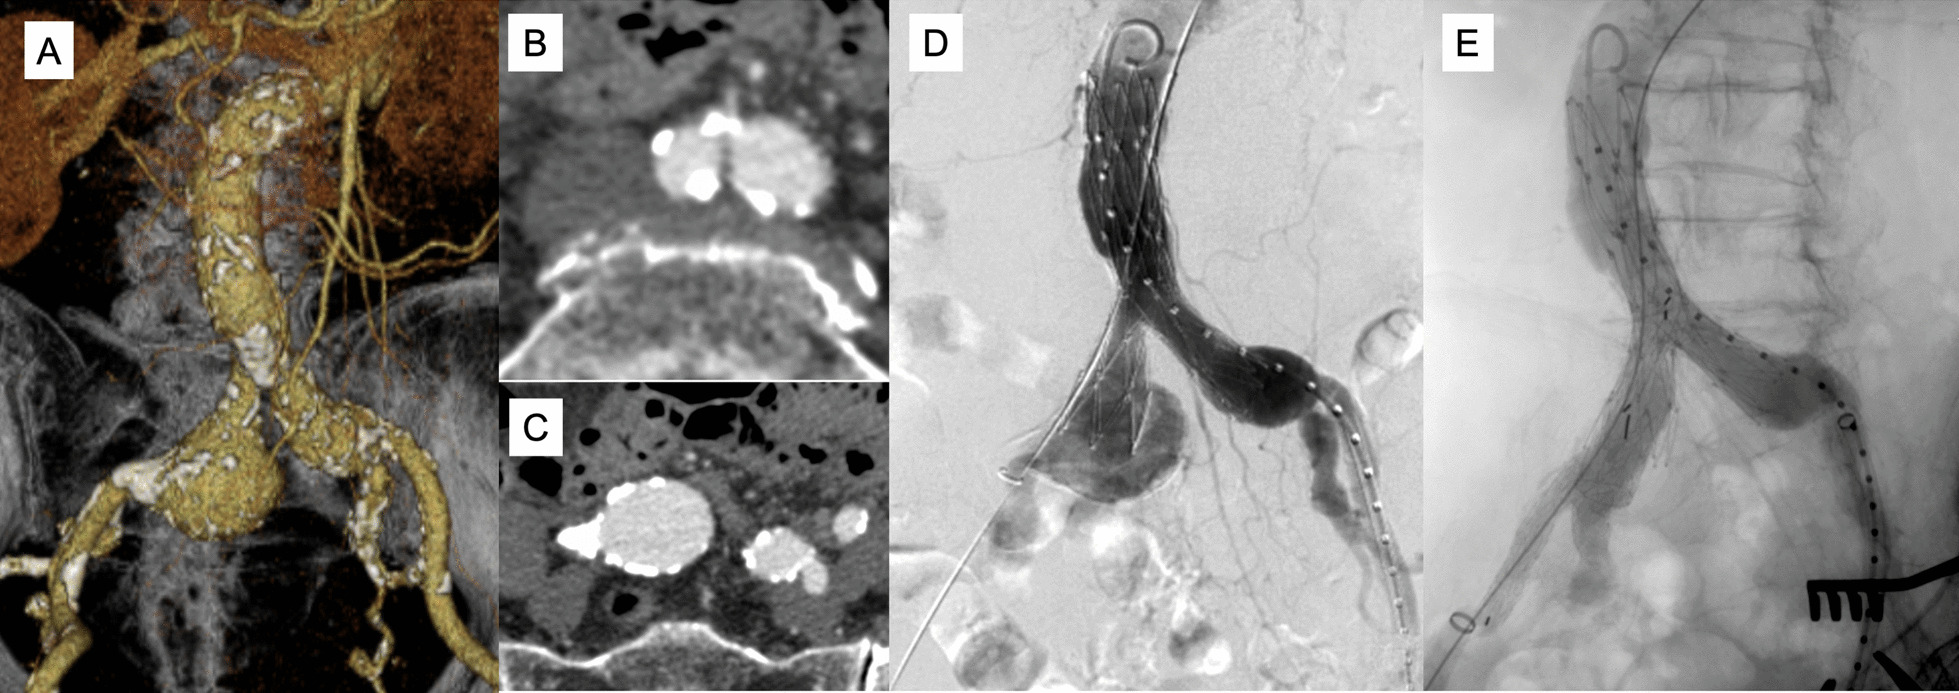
Case 1: A–C Preoperative computed tomography (CT) imaging. A CT angiography (CTA) imaging. B Aorta was not dilated and terminal aorta was narrow. C The proximal portion of right common iliac artery was narrow, measuring 10 mm. D The right common iliac artery aneurysm measuring 38 mm. E–F Intraoperative angiogram. E Aortogram after placement of AFX 2 bifurcated main body. F Completion angiogram showing successful deployment of AFX2 and Iliac branch endoporesis (IBE) and no endoleaks
